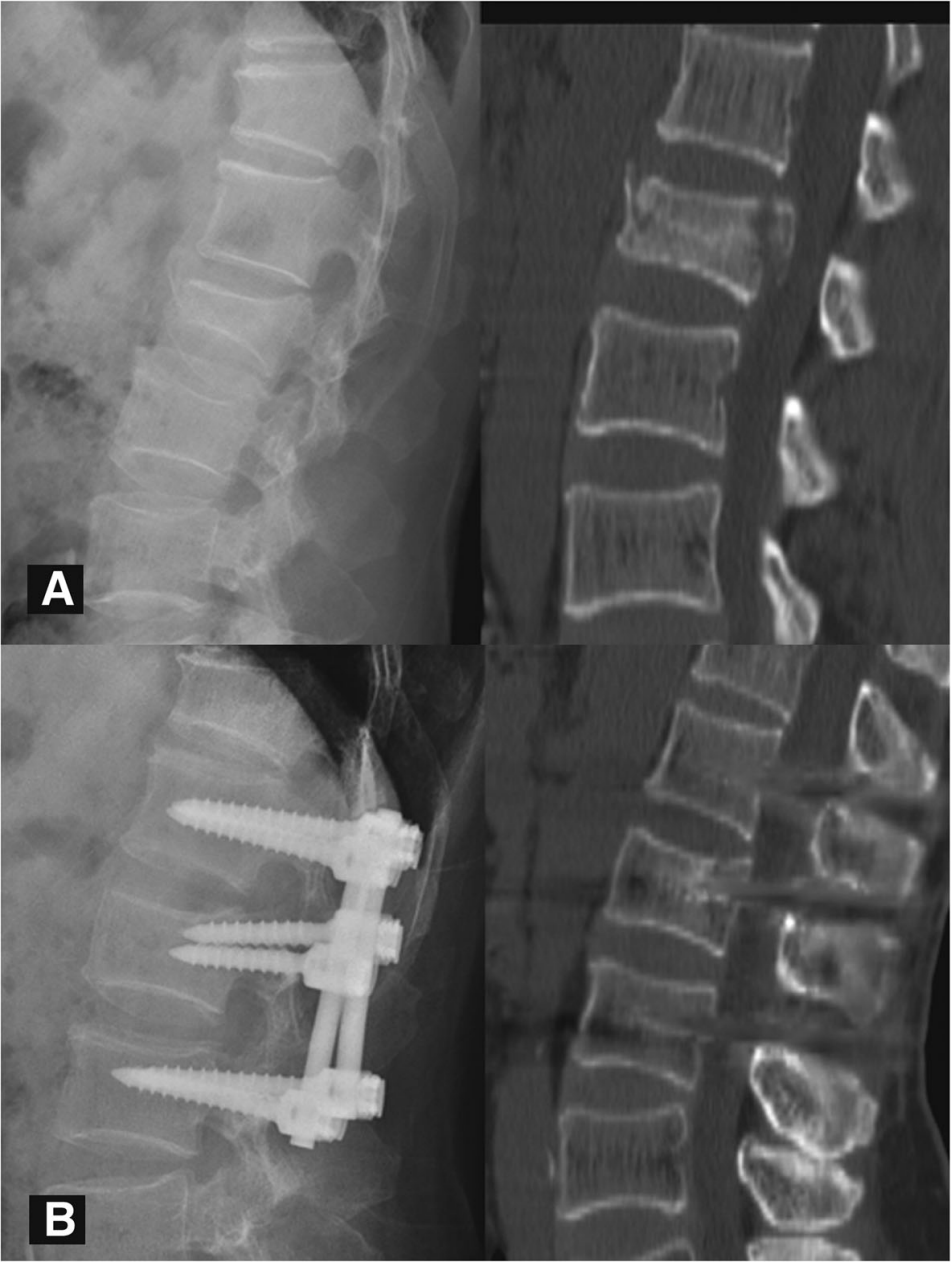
A 53-year-old male had a burst fracture at L2, with an ASIA grade for neurological function of E on admission. a Preoperative X-ray and CT images show an A3 burst fracture. b X-ray and CT images at 15 months after surgery show complete healing of the vertebral body without a cavity, which was classified as HPC type I
